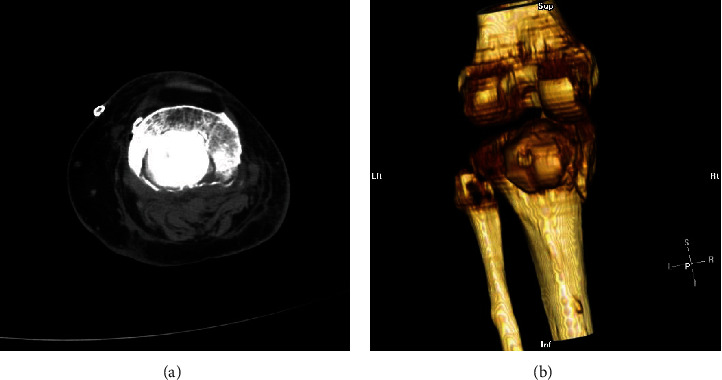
CT-scan ((a) axial, (b) 3-D reconstruction) at six months postoperatively, showing the containment of the PMMA bone cement within the lytic cavity, enabling the patient full weight-bearing.
